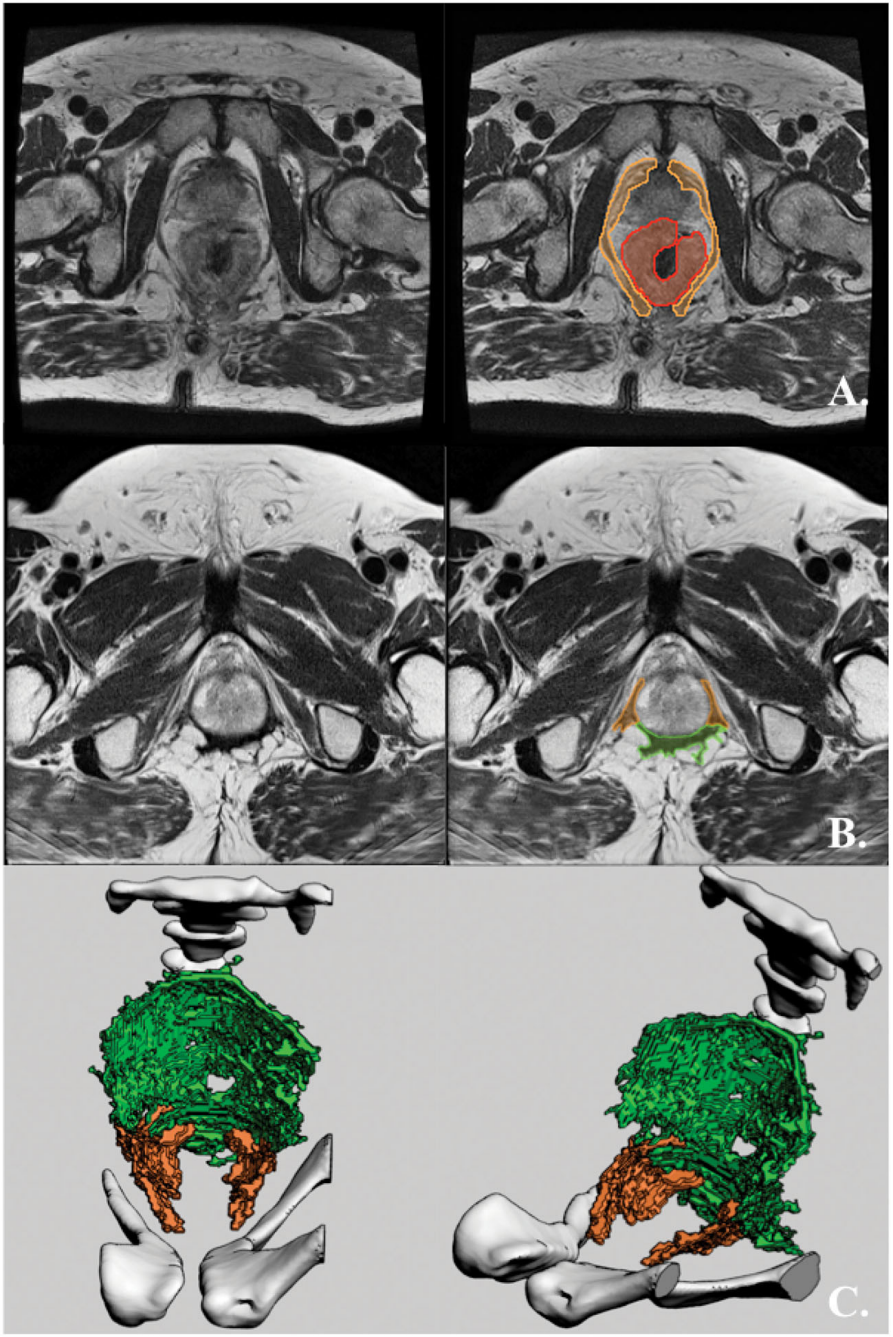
ELAPE without RPD in the posterior quadrants. (A) Preoperative axial T2-weighted MRI of a rectal cancer patient with an mrT3 tumor with circumferential growth (from 1 o'clock to 1 o'clock) and invasive growth from 3 o'clock to 8 o'clock. RPD is hatched in orange, while the tumor is hatched in red. (B) Postoperative axial T2-weighted MRI of the same patient. RPD hatched in orange while supporting mesh hatched in green. (C) The pelvic diaphragm of the same patient, 3D-rendered and shown in superior and antero-lateral superior views. The rendering is made from the above postoperative MRI. The bony pelvis is rendered in white, RPD is rendered in orange, and supporting mesh is rendered in bright green.
